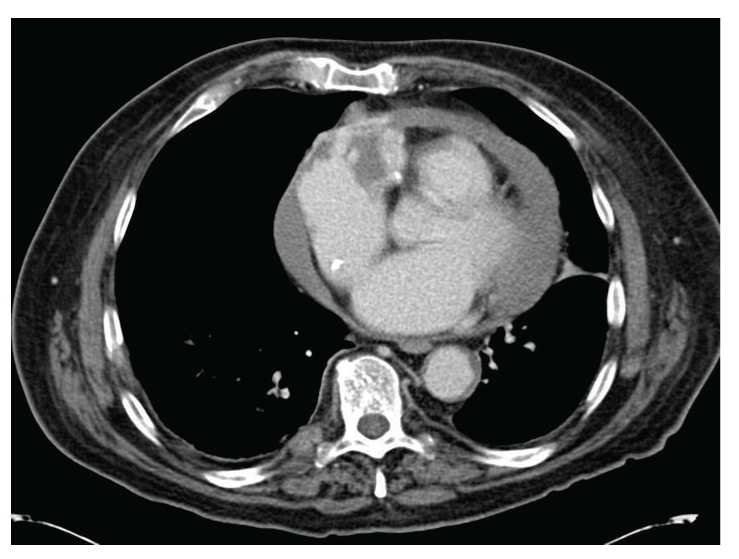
Computed tomography scan of the chest showing the right atrial mass and pericardial effusion.
